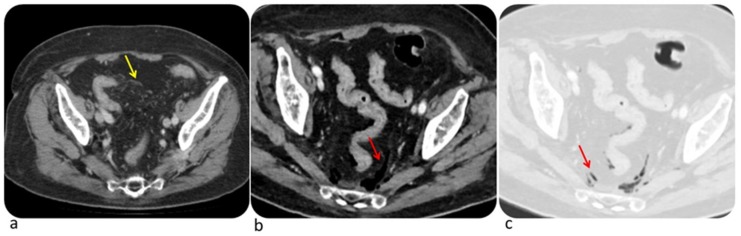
Complicated immune-related colitis in a 54-year-old man with metastatic melanoma treated first with ipilimumab then with Pembrolizumab. CT image shows mesenteric vessel engorgement (a, yellow arrow); CT performed two months later demonstrates free intraperitoneal air (b,c, red arrow) due to intestinal perforation. © Department of Radiology, Candiolo Cancer Institute—IRCCS. Turin.
